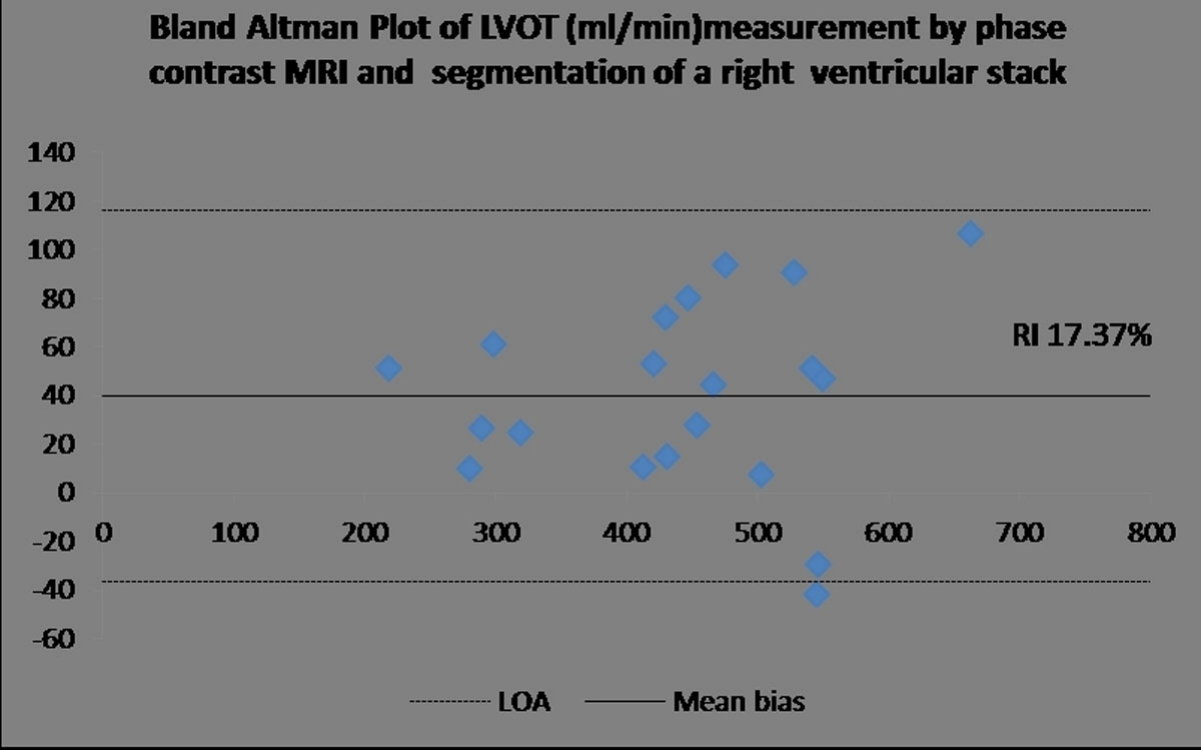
# Validation of MRI quantification of biventricular cardiac function in term and preterm neonates
